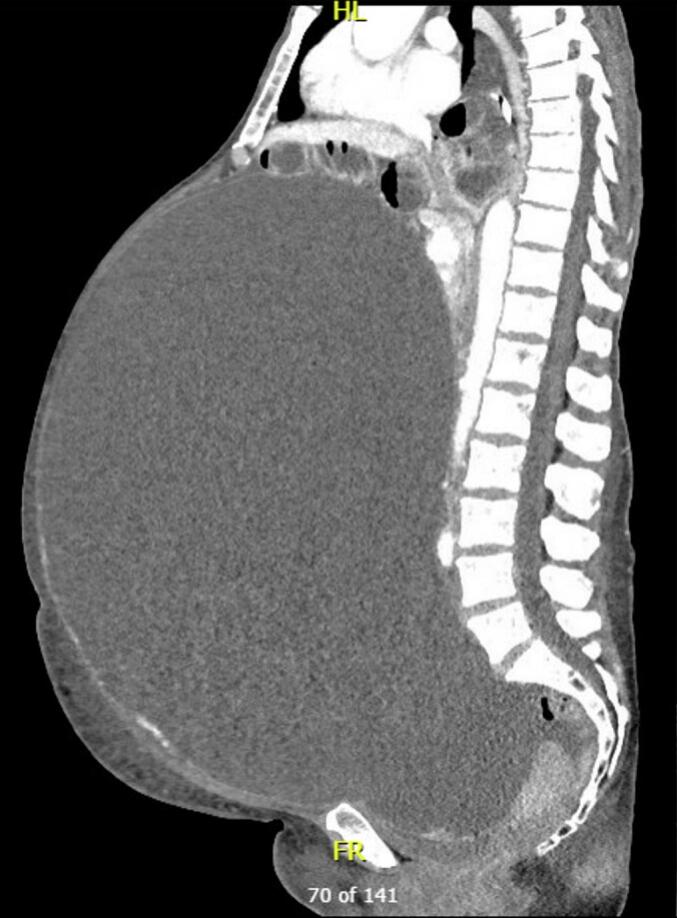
CT depicting a massive right ovarian lesion with displacement of bowel.
